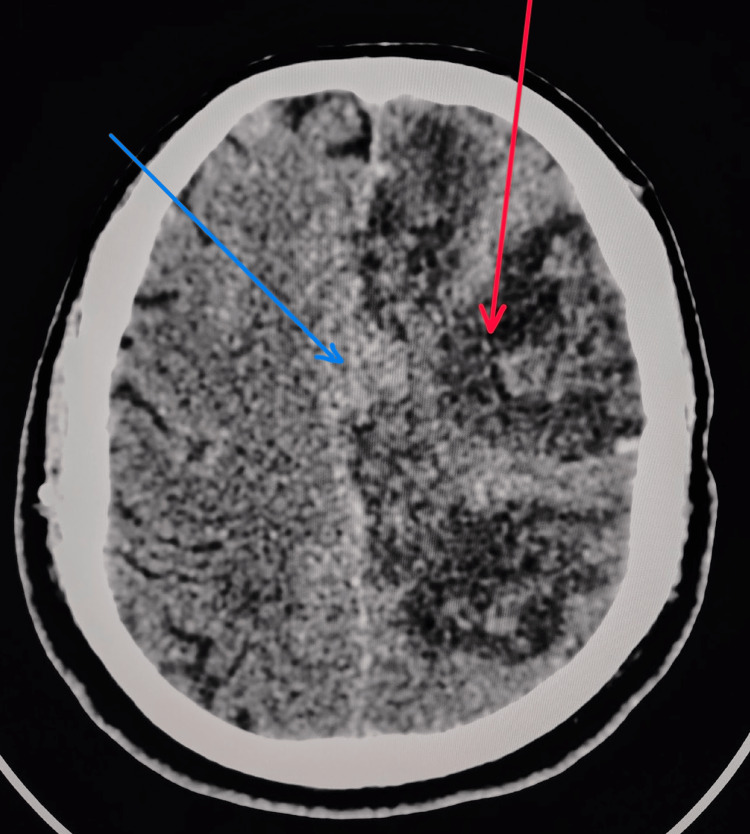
Repeat non-contrast CT of the brain showing hemorrhagic transformation (red arrow) of the infarct with a midline shift (blue arrow) of 7.5 mm, following clinical deterioration after 48 hours.
